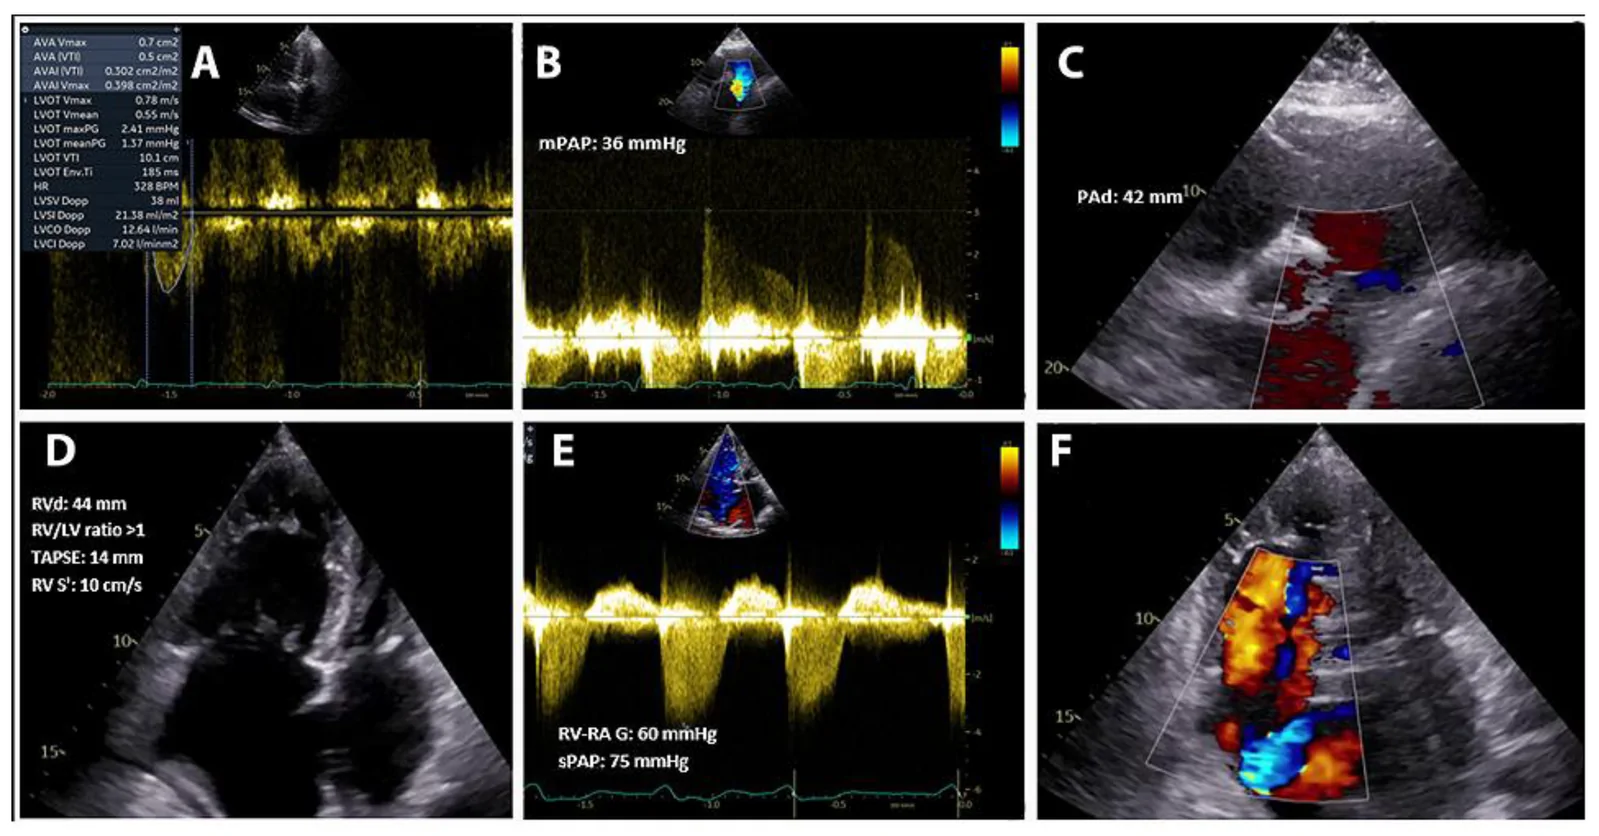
Post-decongestion echocardiographic reassessment. (A) Degenerative aortic valve disease with severe aortic stenosis (Vmax 4.7 m/s, mean gradient 45 mmHg, AVA 0.53 cm^2^, AVA index 0.29 cm^2^/m^2^) and mild regurgitation; (B) High probability of pulmonary hypertension (non-invasive mean pulmonary artery pressure of 36 mmHg determined using CW doppler early diastolic velocity gradient); (C) Moderate pulmonary regurgitation with dilatation of the pulmonary artery (PA diameter 42 mm); (D) Dilated right ventricle (RV diameter 44 mm, RV/LV ratio >1) with significant RV systolic dysfunction (TAPSE 14 mm, RV s′ 10 cm/s); (E) Severe pulmonary hypertension, demonstrated by consistently increased RV-RA gradient; (F) Severe tricuspid regurgitation on color doppler interrogation.
